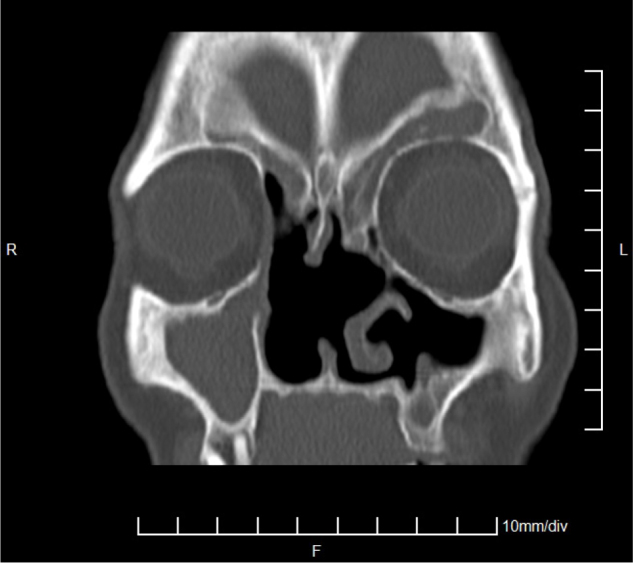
CT scan of the sinuses – 12 months after surgery, coronal plane.
